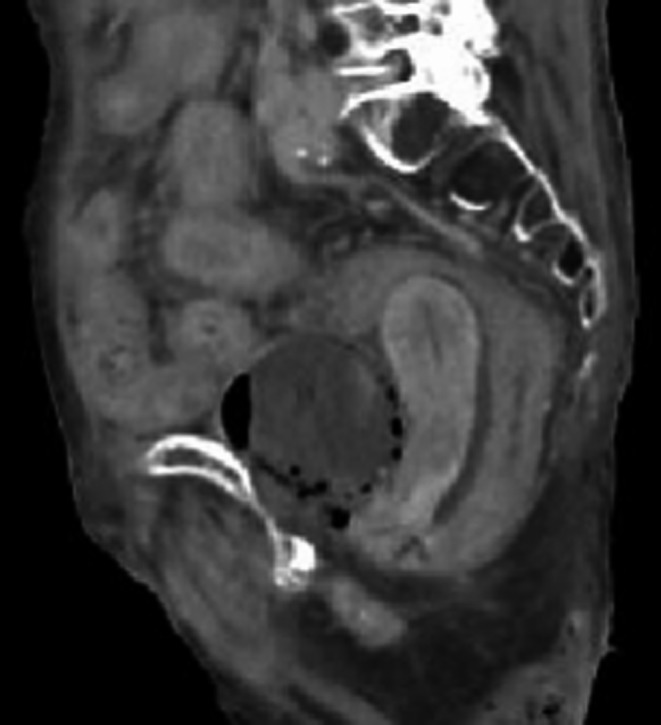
Computed tomography of the abdomen and pelvis shows diffuse gas collection within the urinary bladder wall (Sagittal plane)
